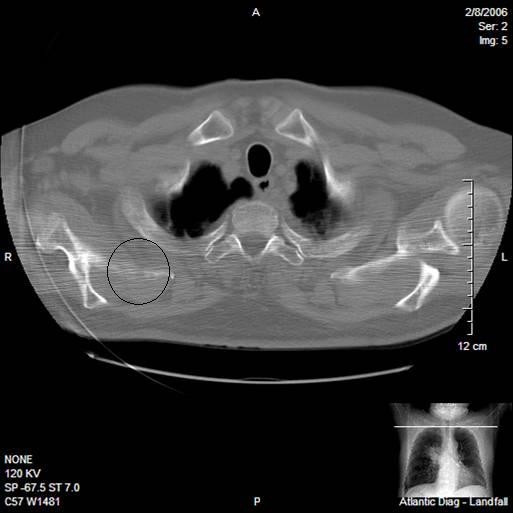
CT of the chest reveals cortical lucency, expansile destruction, and medullary invasion due to metastatic lung carcinoma affecting the right scapula.
